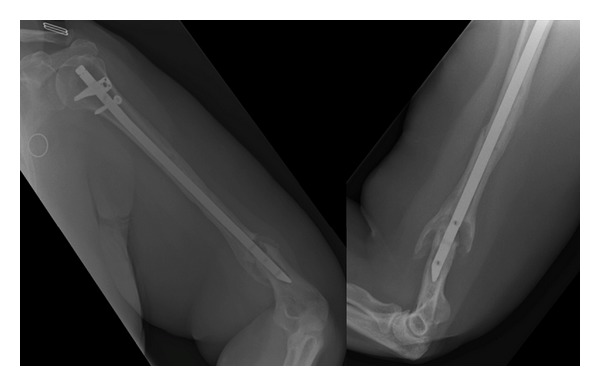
AP and lateral radiographs demonstrating hypertrophic nonunion of distal humeral periprosthetic fracture.
